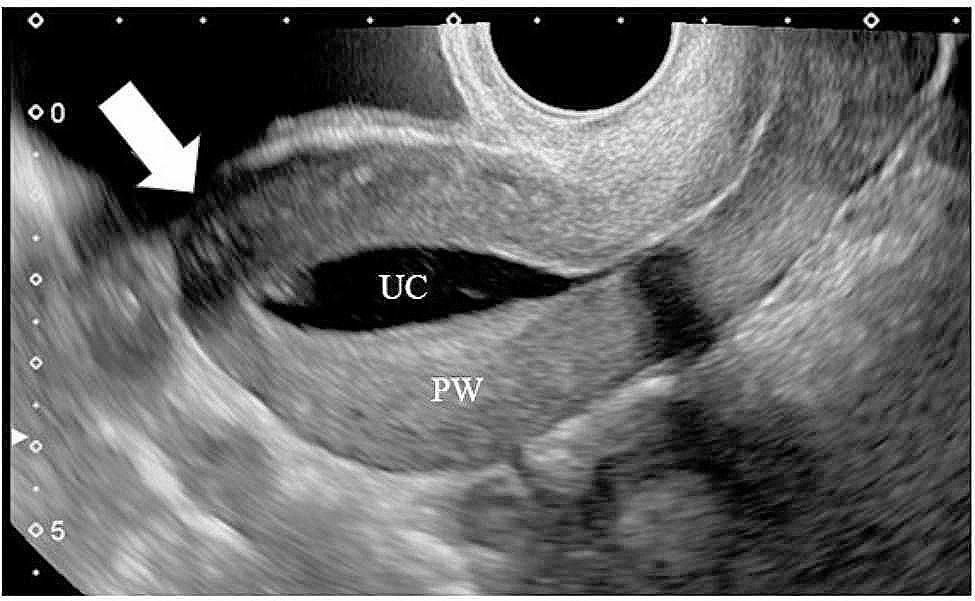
Examination of the scar 12 months after transverse uterine fundal incision by sonohysterogram While the uterine cavity was expanded with a saline injection, the scar area was observed by transvaginal ultrasonography Arrow: the transverse uterine fundal incision scar UC: uterine cavity filled with saline PW: posterior wall of the uterus
